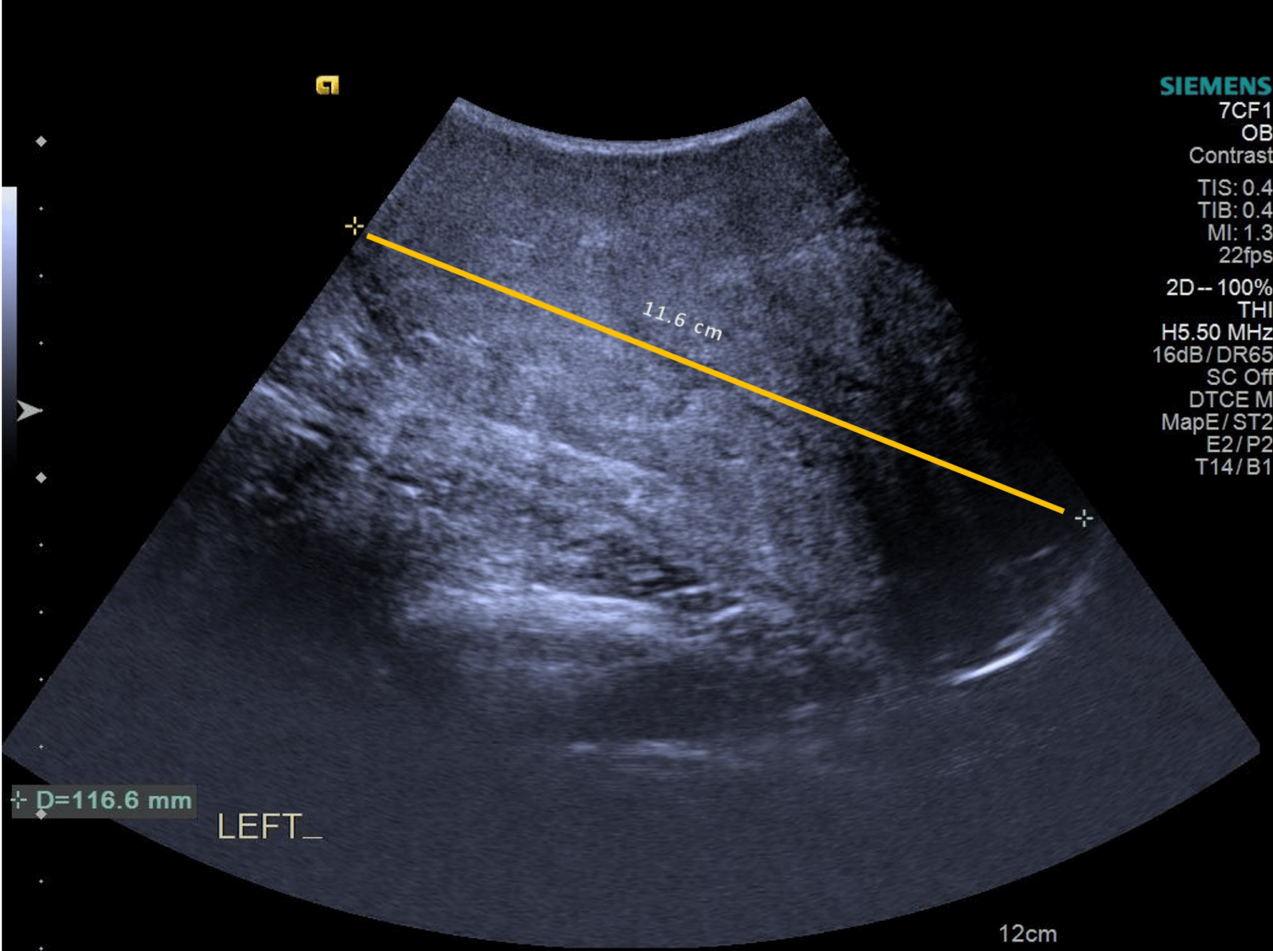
A large field-of-view ultrasound image demonstrating a 11.6-cm heterogeneous hypoechoic solid mass in the left breast.
